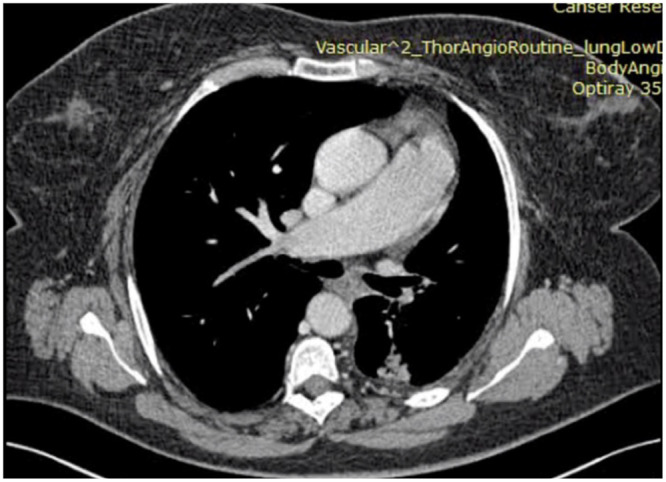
Preoperative computed tomography scan showed a unilateral absence of the left pulmonary artery.
